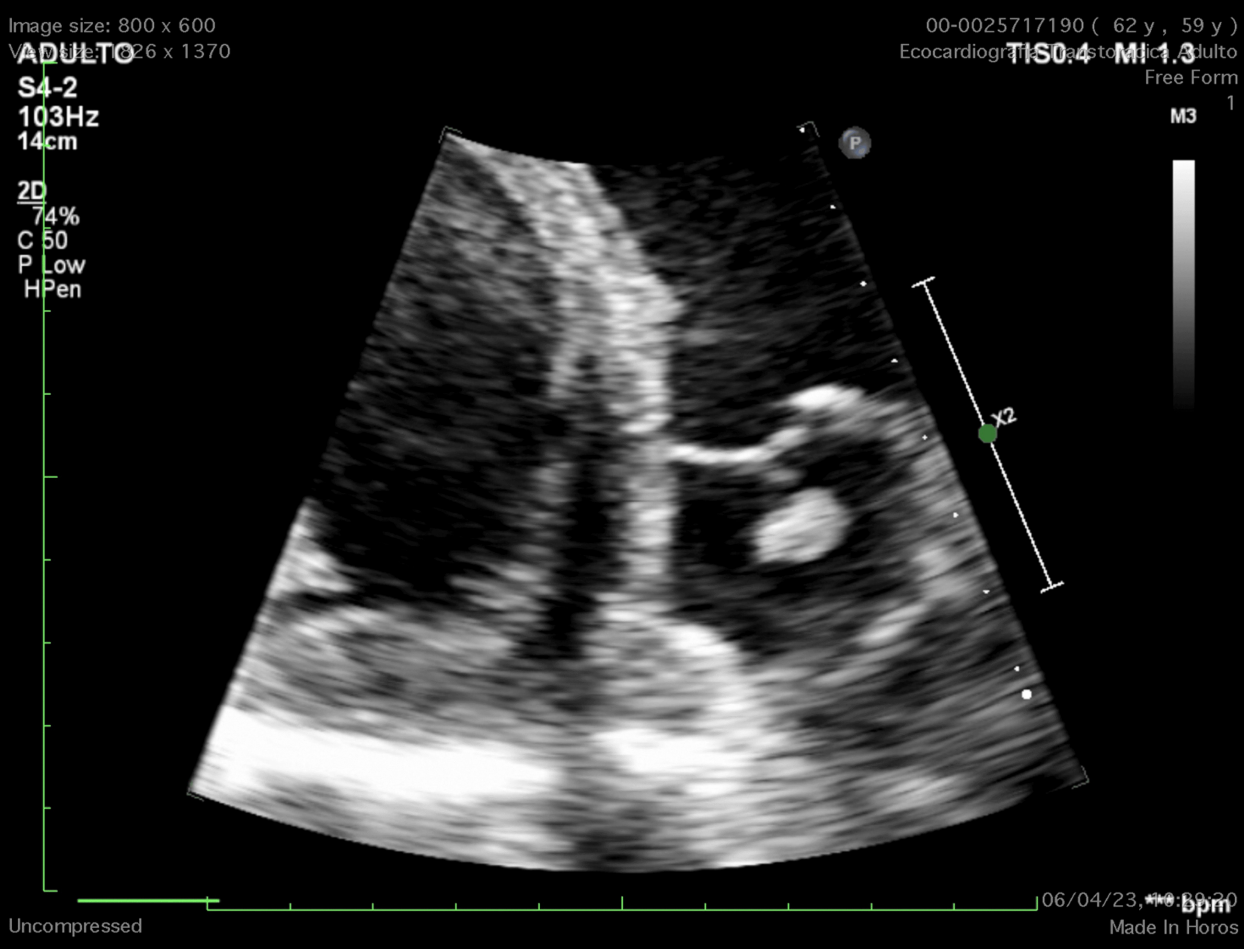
Transthoracic echocardiogram, parasternal short-axis view, showing a papillary fibroelastoma attached to the coaptation surface of the pulmonary valve (zoomed view).
